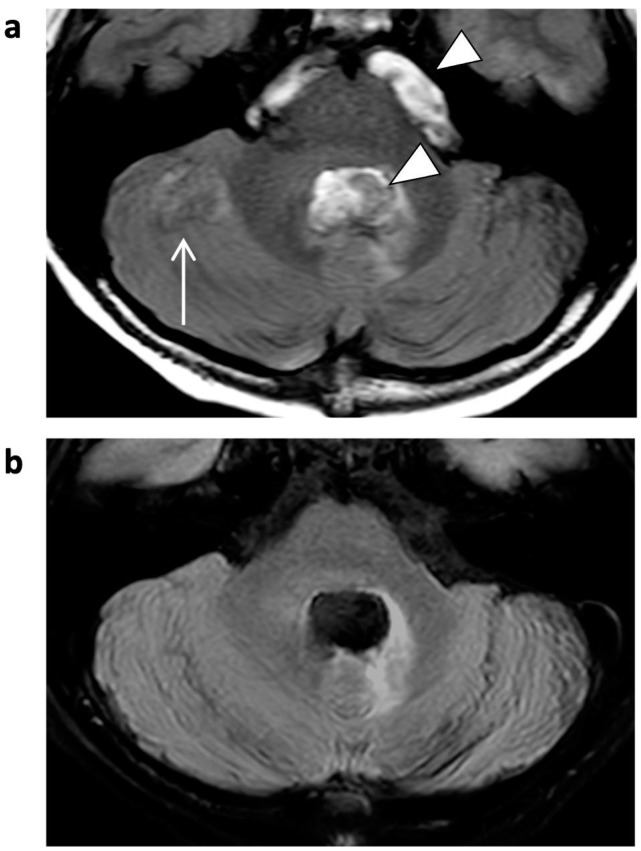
FLAIR images of a 5-year-old male patient with astrocytoma (post-resection). Bright CSF-flow-related enhancement (FRE, arrowheads) in fourth ventricle and prepontine cisterns is seen in SENSE FLAIR (a), not present in CS FLAIR (b). As a consequence, the CSF-dependent ghosting artifacts (arrow) did not occur.
